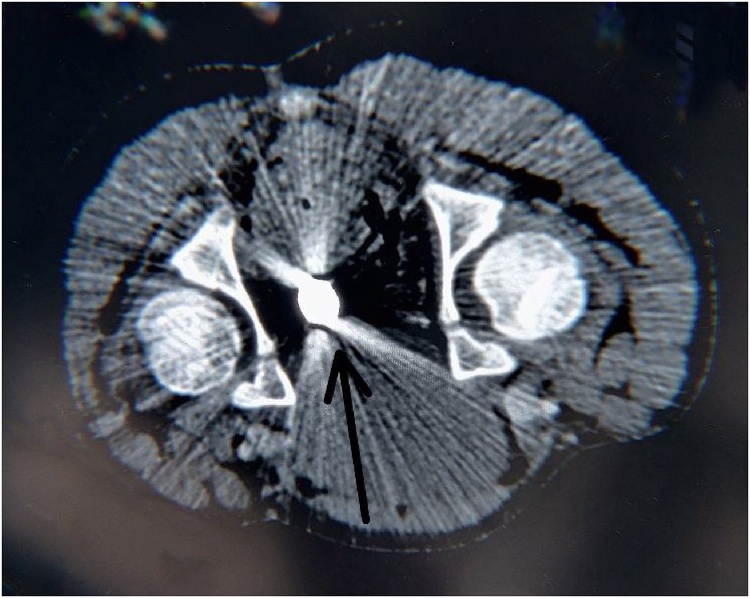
CT scan (transverse section) showing foreign body (rod) posterior to Urinary bladder and anterior to rectum.
